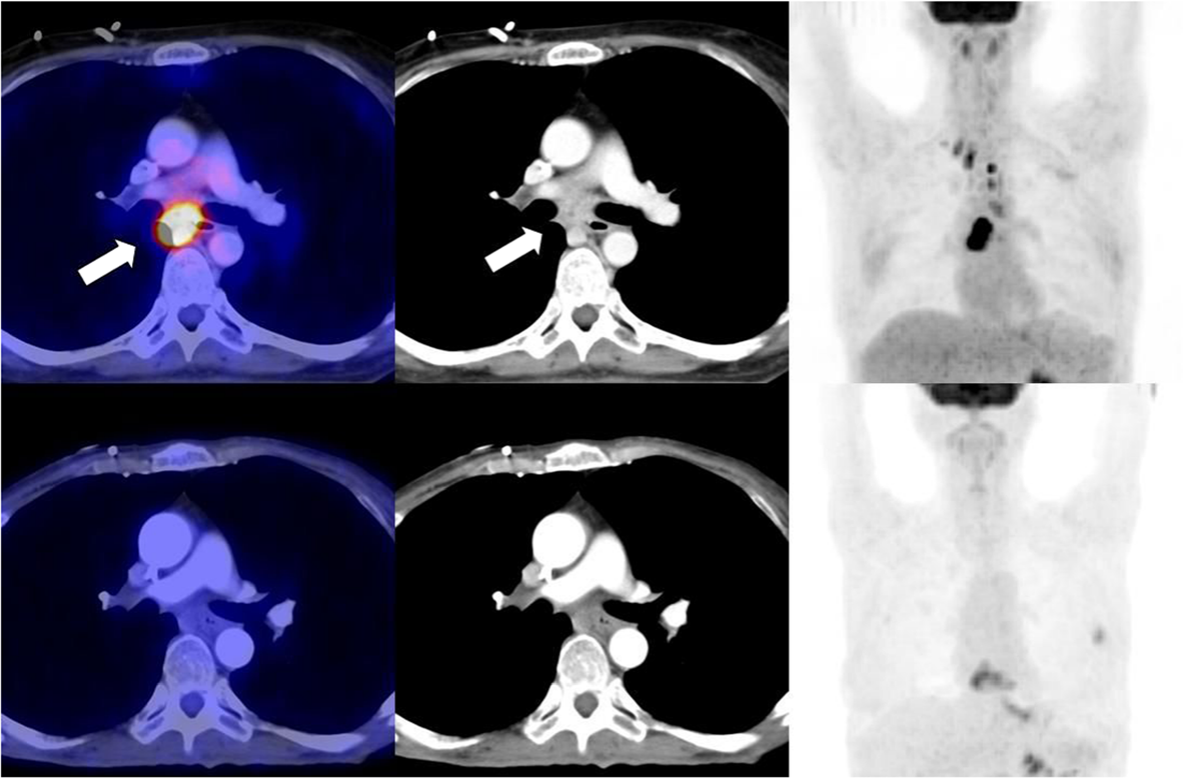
Patient 1. Upper row - before tuberculostatic therapy: High FDG uptake in an infracarinal lymph node (left panel: fusion images of PET/CT) correlating with central necrosis in the contrast enhanced CT (middle panel). Coronal MIP with high FDG uptake in several mediastinal lymph nodes (right panel). Lower row - 8 month follow up: No FDG uptake (left panel: PET/CT) and significant size reduction of the visualized lymph nodes (middle panel: ceCT). No pathological FDG uptake in the mediastinal lymph nodes on coronal MIP of PET (right panel).
